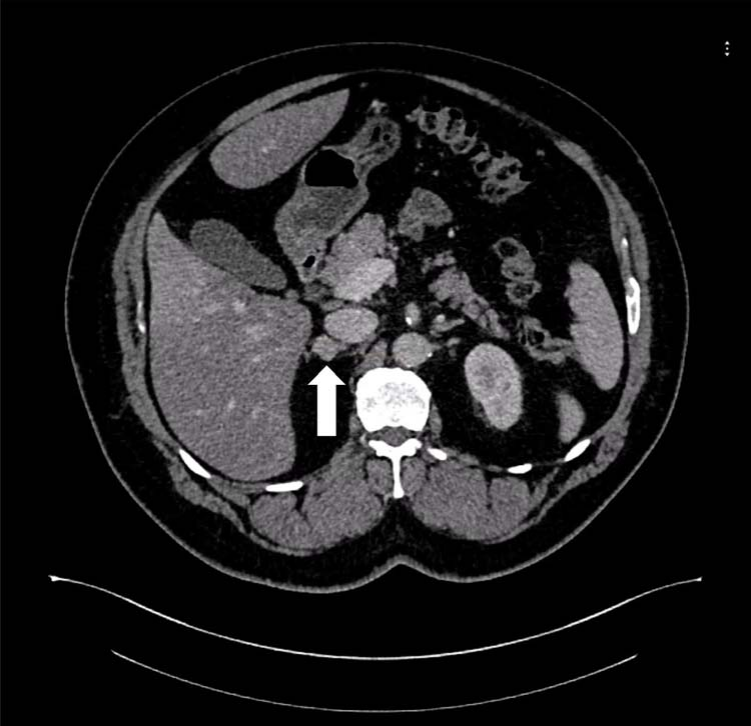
Axial CT of the abdomen showing the right adrenal mass in Patient 2 (white arrow).
